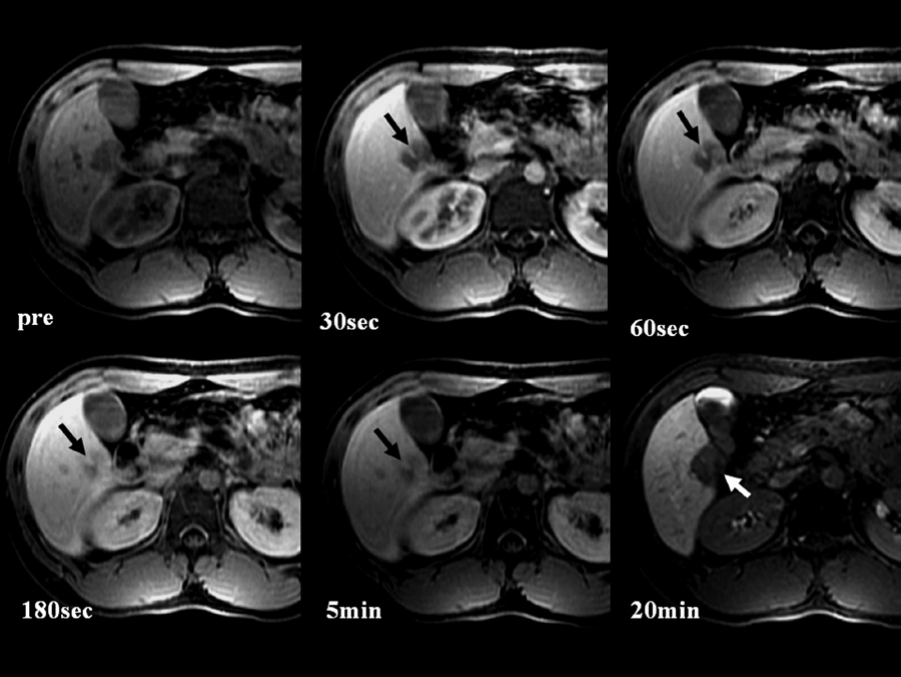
Gadoxetic acid (Gd-EOB-DTPA)–enhanced magnetic resonance images, contrast-enhanced T1-weighed image shows the following: low signal intensity hepatic nodule on the precontrast T1-weighted image (upper left), showing peripheral septa-like enhancement (black arrow) in the arterial phase (upper middle), and more globular centripetal enhancement during the portal venous phase (upper right), the equilibrium phase (lower left) to 5 minutes delayed phase (lower middle), and became low signal intensity on the hepatobiliary phase (lower right) with focal capsular retraction (white arrow).
